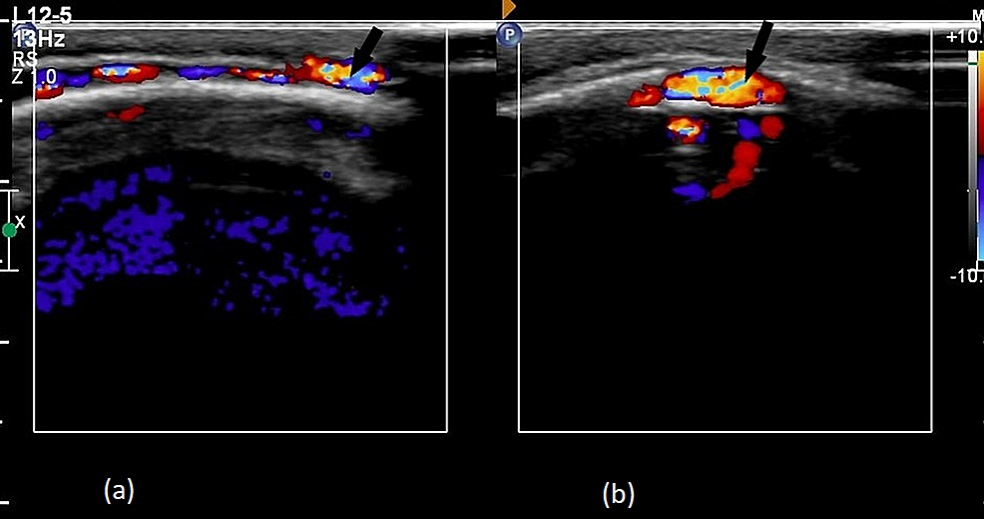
Color Doppler ultrasound showing slow venous flow in extracranial vessels in parietal region (black arrows) (a), in direct communication with superior sagittal sinus (b).
